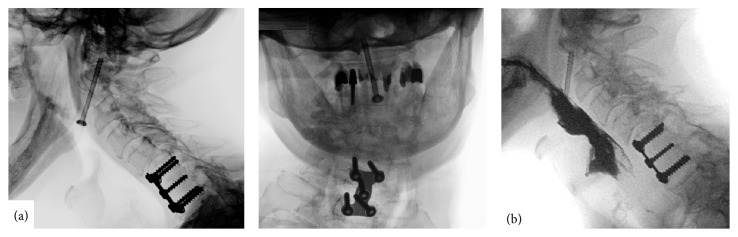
(a) X-ray imaging disclosed a ventral migration of the odontoid screw, 8 years after operation, lateral view (left) and a.p. view (right). (b) Pharyngeal stenosis was excluded by fluoroscopic swallow examination, lateral view.
